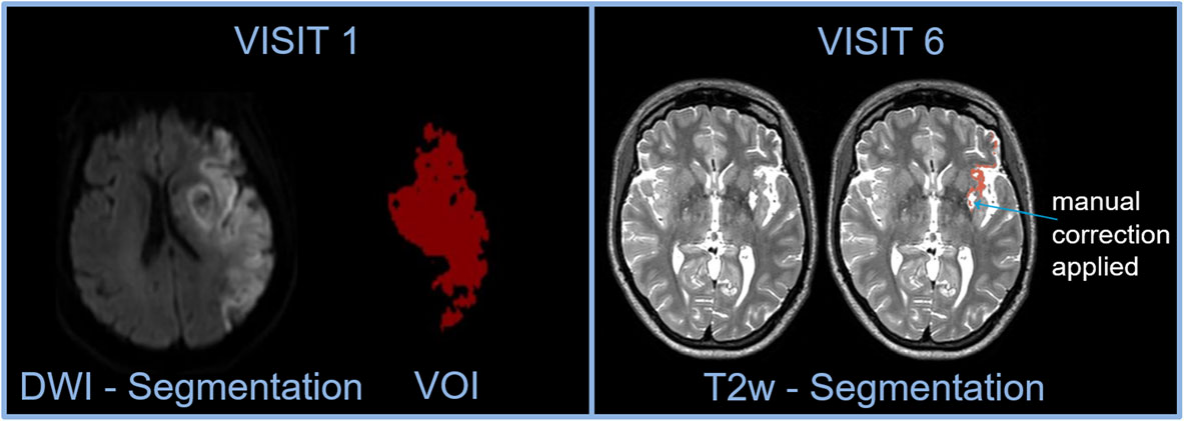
Assessment of primary endpoint. Infarct volume is manually segmented on DWI (diffusion weighted imaging) MRI scans at visit 1, i.e. after potential lysis therapy and before ASV-treatment, and on T2w MRI scans at visit 6. The lesion mask (volume of interest, VOI) is used to preselect the lesion on the T2w images and manual correction is then applied
